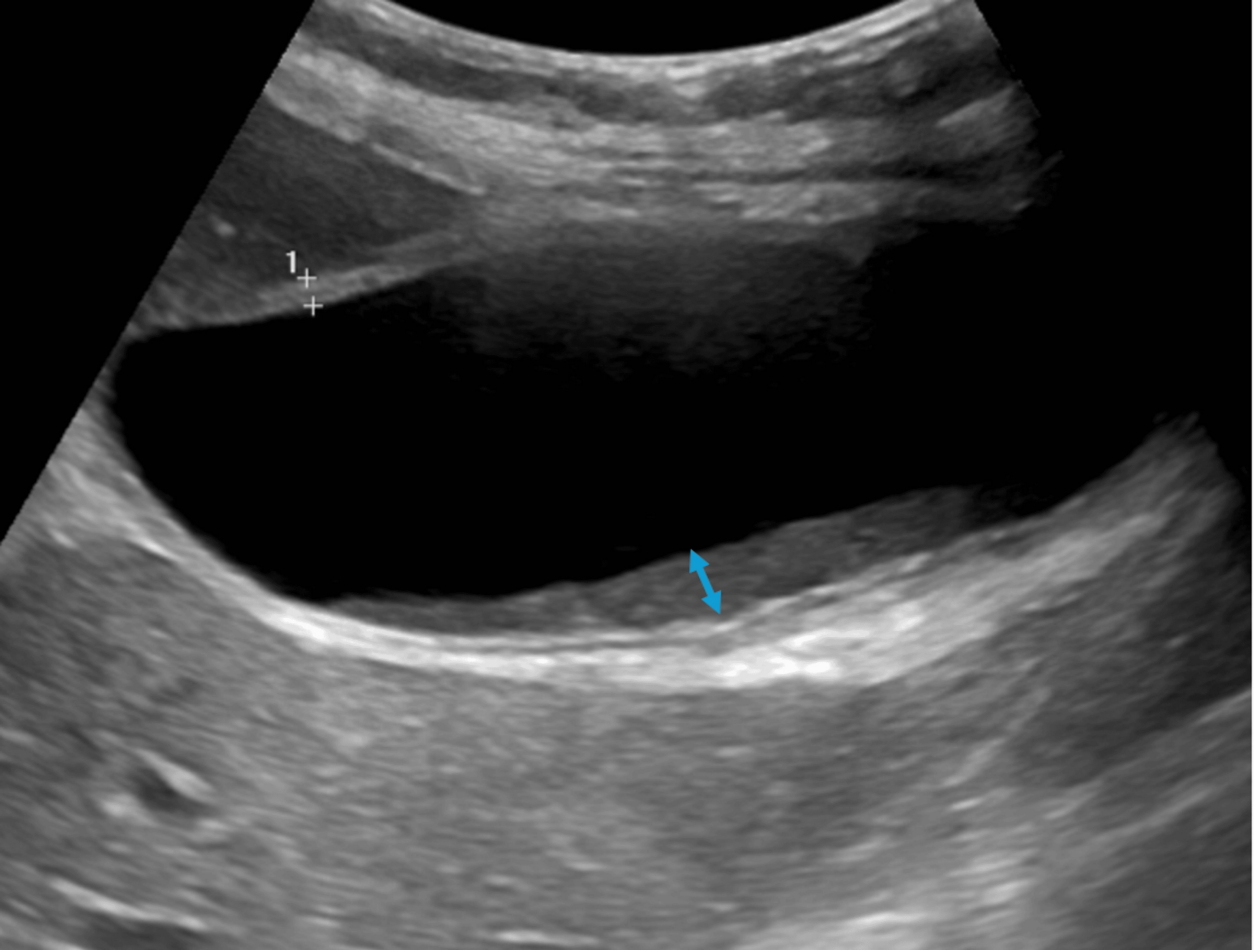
Gallbladder sludge. Right upper quadrant point-of-care ultrasonography was performed using a curvilinear transducer in the longitudinal orientation, demonstrating a distended gallbladder with a thickened wall (calipers). Dependent intraluminal echogenic material, consistent with biliary sludge (double-headed blue arrow), was also observed.
